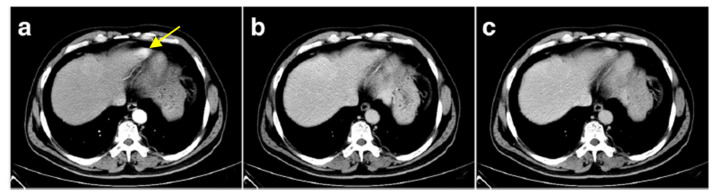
Contrast enhanced liver CT. (a–c): Arterial, portal, and equilibrium phase, respectively (reprinted from [55] under the license CC BY 4.0). Yellow arrow: left liver apex mass which re-enhanced contrast during the arterial phase.
